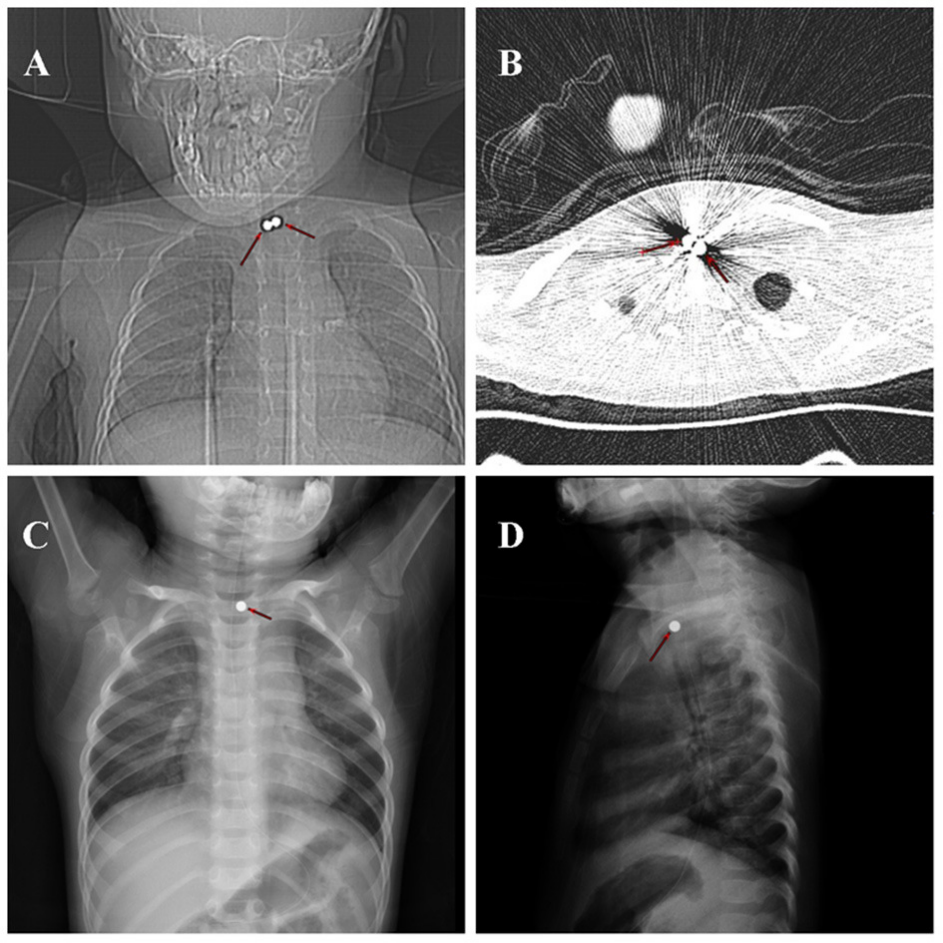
Location mapping and horizontal CT of the trachea and bronchi revealed two round high-density shadows (red arrow) of diameter ~0.5 cm that appeared to be located in the upper part of the trachea at the level of the chest (A,B). A residual round, metallic, foreign body shadow was apparent at the entrance of the thoracic cage on chest frontal and lateral DR performed at 6 h post-operatively (C,D).
